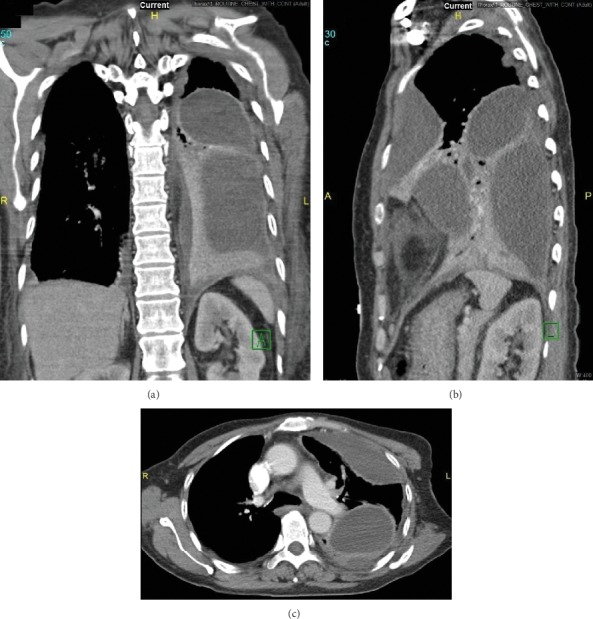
Chest CT images of the multiloculated empyema. Coronal view (a), sagittal view (b), and axial view (c).
